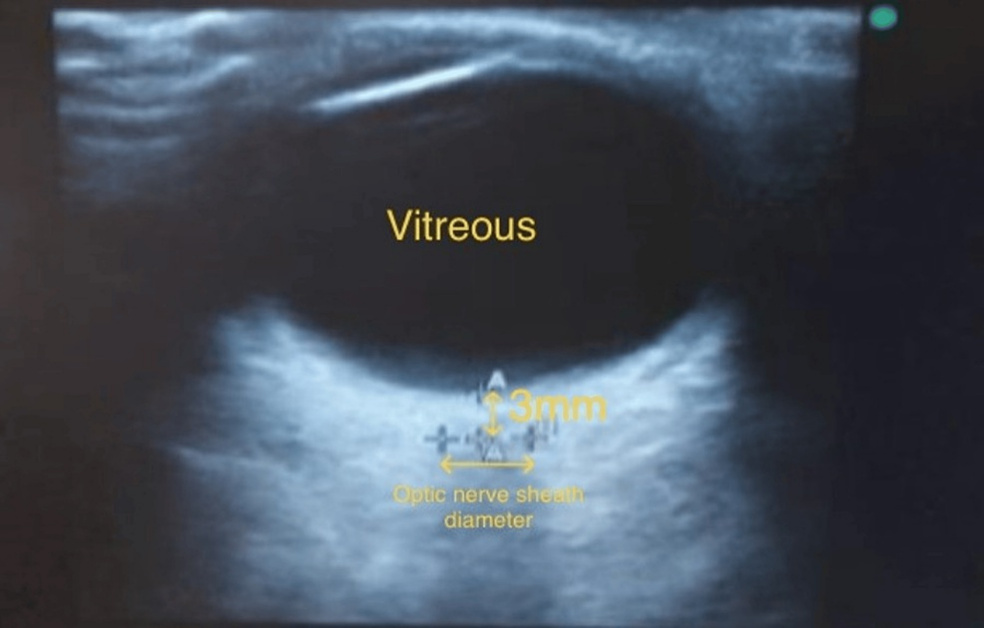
Measurement of the optic nerve sheath diameter by ultrasound.
